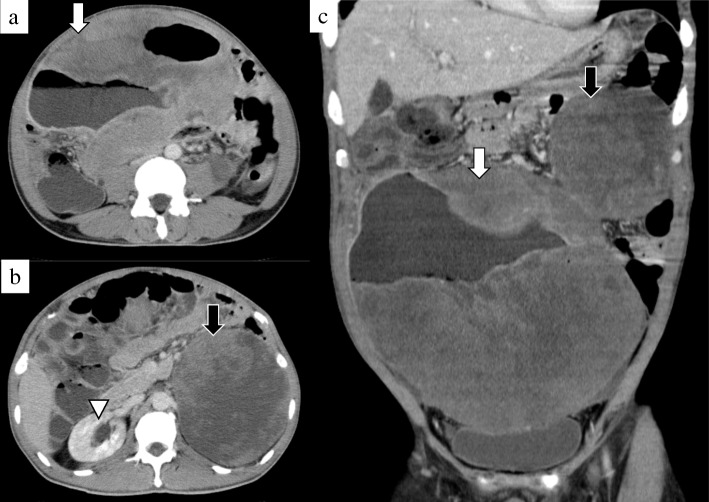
An abdominal enhanced computed tomography scan. a An intra-abdominal mass with a diameter of 230 mm (white arrow). The dorsal part of the intra-abdominal tumor contained liquid and air, which indicated abscess formation. b A retroperitoneal mass under the left kidney with a diameter of 146 mm (black arrow), intra-abdominal tumor compressed the right ureter, and the right kidney was in hydronephrosis state (white arrowhead). c Both tumors had internal inhomogeneity, and the upper part of the intra-abdominal tumor would contain abscess (white arrow, intra-abdominal tumor; black arrow, retroperitoneal tumor)
